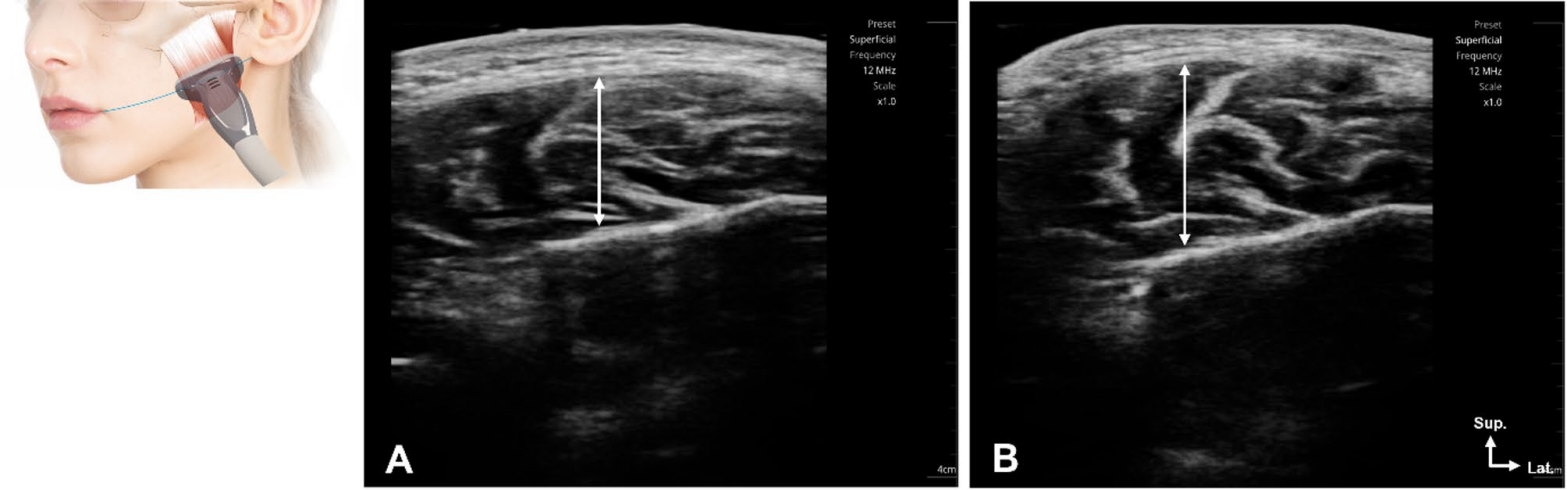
Ultrasound examination guidelines for measuring masseter muscle thickness. (A) Measurement in the resting state. (B) Measurement during maximal voluntary clenching. The probe was placed perpendicular to the skin surface at the midpoint between the zygomatic arch and the mandibular angle. Sup, superficial; Lat., lateral
